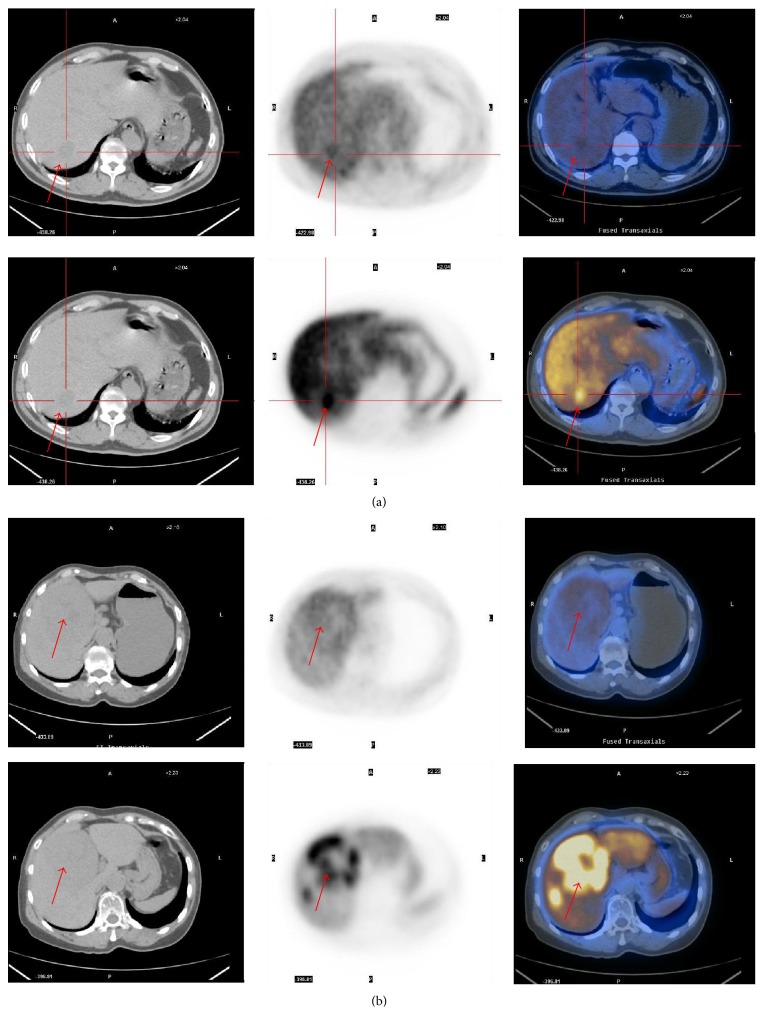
(a) ^18^F-FDG PET/CT images showed that lesion in the right hepatic lobe was ^18^F-FDG positive (SUV_max_: 2.4), and ^11^C-CHO PET/CT images showed that the uptake of lesion was increased (SUV_max_: 10.7) (red arrows on a). (b) ^18^F-FDG PET/CT image showed that lesion in the right hepatic lobe was ^18^F-FDG negative; ^11^C-CHO PET/CT image showed that the uptake of lesion was increased (SUV_max_: 12.8). These two cases in the right hepatic lobe were consistent with PHC (red arrows on b).
